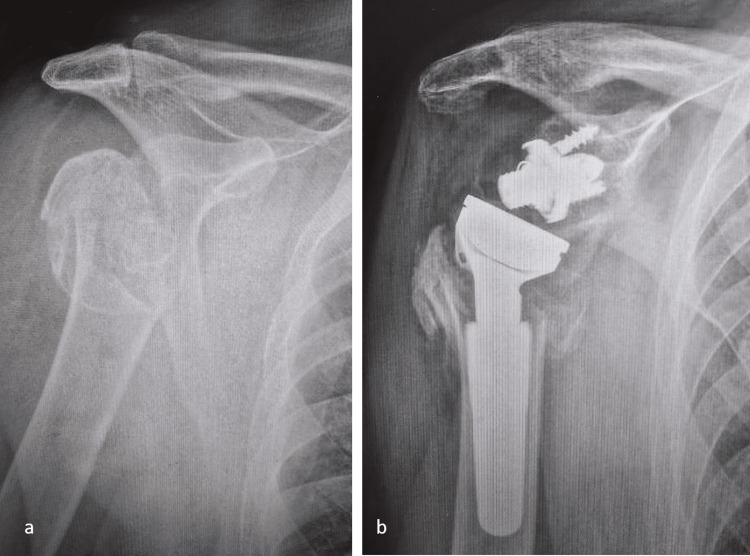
Comminuted proximal humerus fracture managed with reverse TSA (a) Preoperative radiograph demonstrating a severely comminuted humeral head fracture associated with posterior instability. (b) Postoperative radiograph showing a reverse TSA performed for reconstruction and stability. TSA, total shoulder arthroplasty
